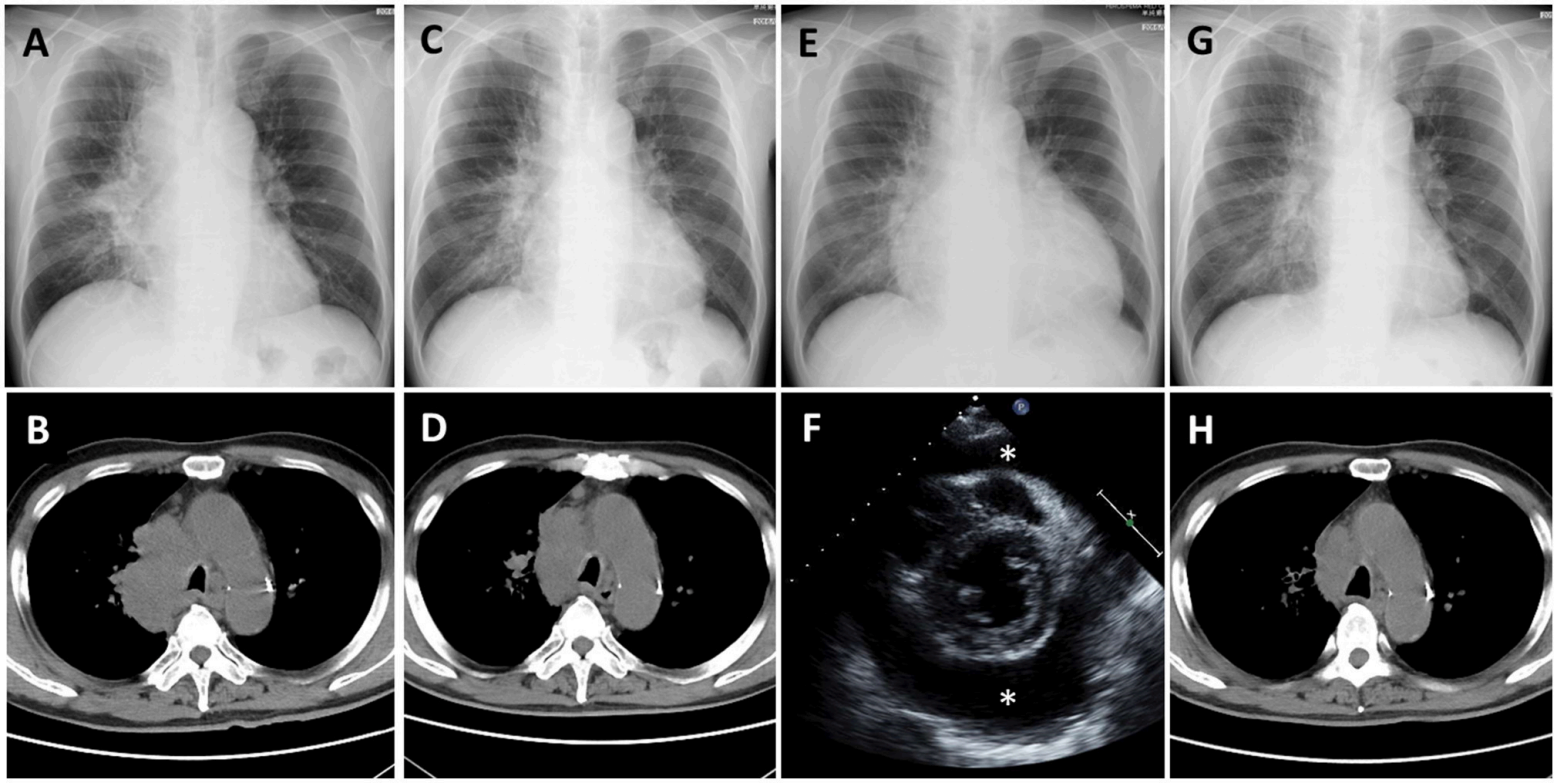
Case 1 (A,B) Chest X-ray and computed tomography (CT) before nivolumab administration shows a lung mass from the right mediastinum to the right hilar region. (C,D) Chest X-ray and CT after 2 cycles of nivolumab administration shows tumor regression. (E) Chest X-ray after 4 cycles of nivolumab administration shows cardiomegaly. (F) Echocardiography shows massive pericardial effusion (*: effusion). (G,H) Chest X-ray and CT after pericardiocentesis followed by nivolumab administration shows improvement of cardiomegaly and further tumor regression.
